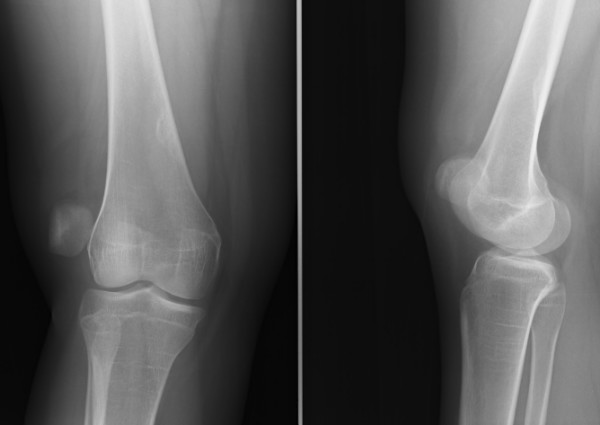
Pre-operative radiograph of the right knee showing patellar dislocation.
